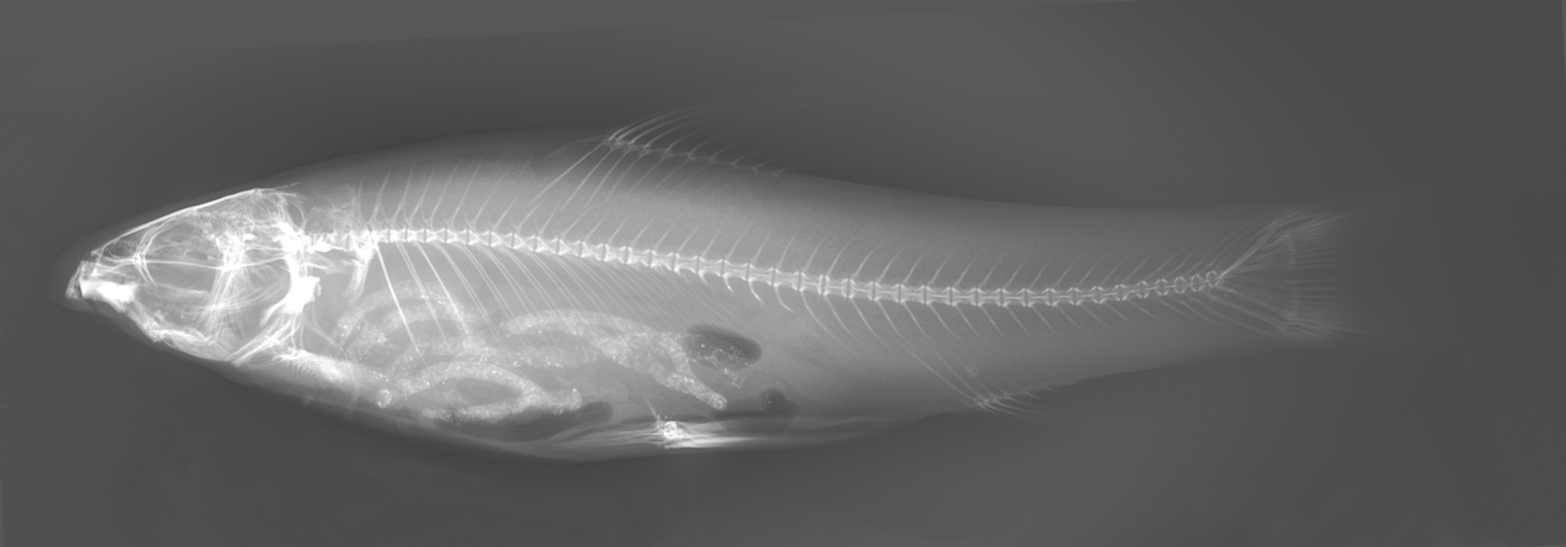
Radiograph of *Zuojiangia jingxiensis*, paratype, KIZ 2012005694, 93.6 mm SL.
